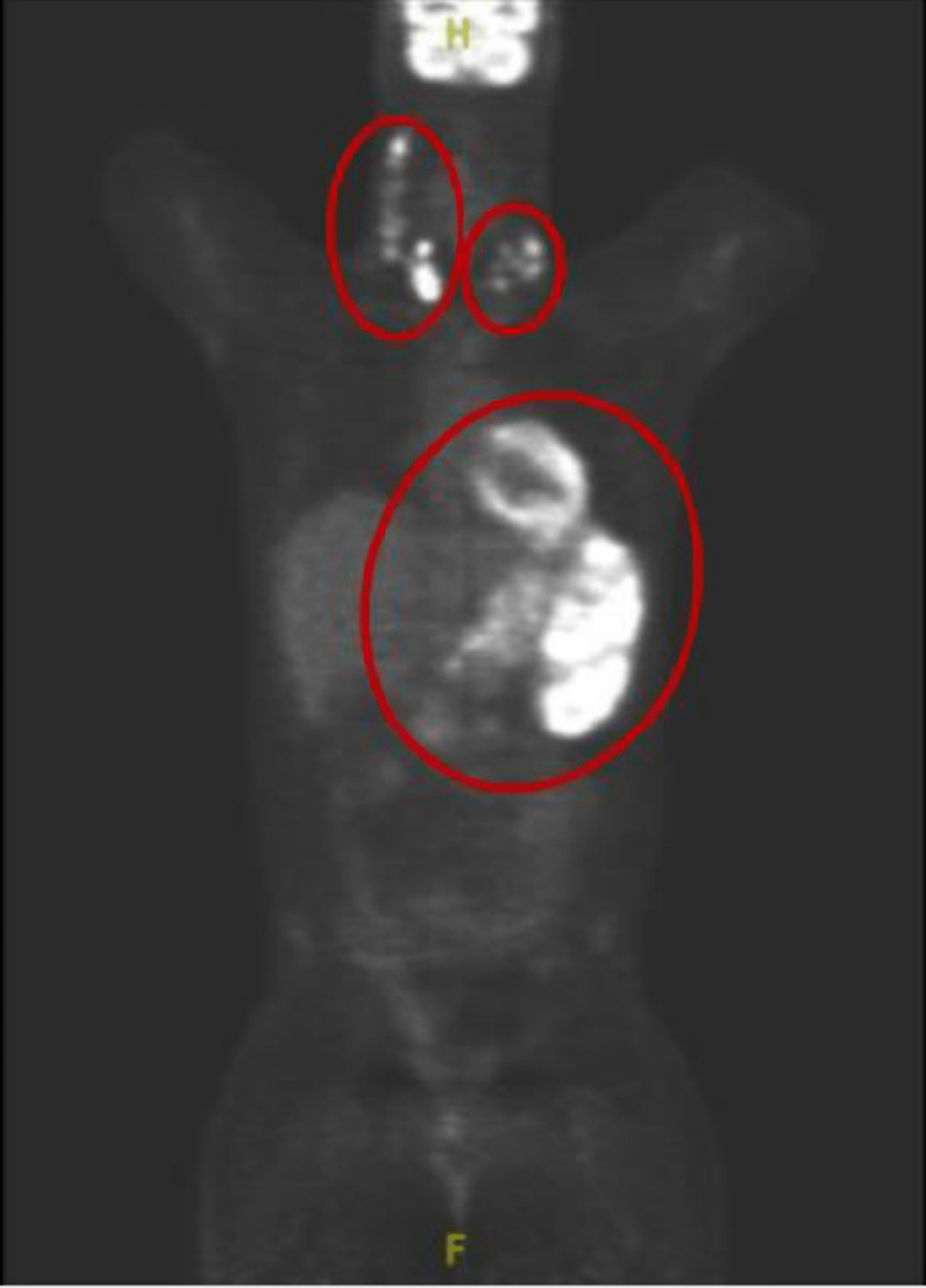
Positron emission tomography. Coronal plane image of positron emission tomography/computed tomography scan taken before treatment. The image shows adenopathy activity above and below the diaphragm with splenic infiltration encased in red circles.
